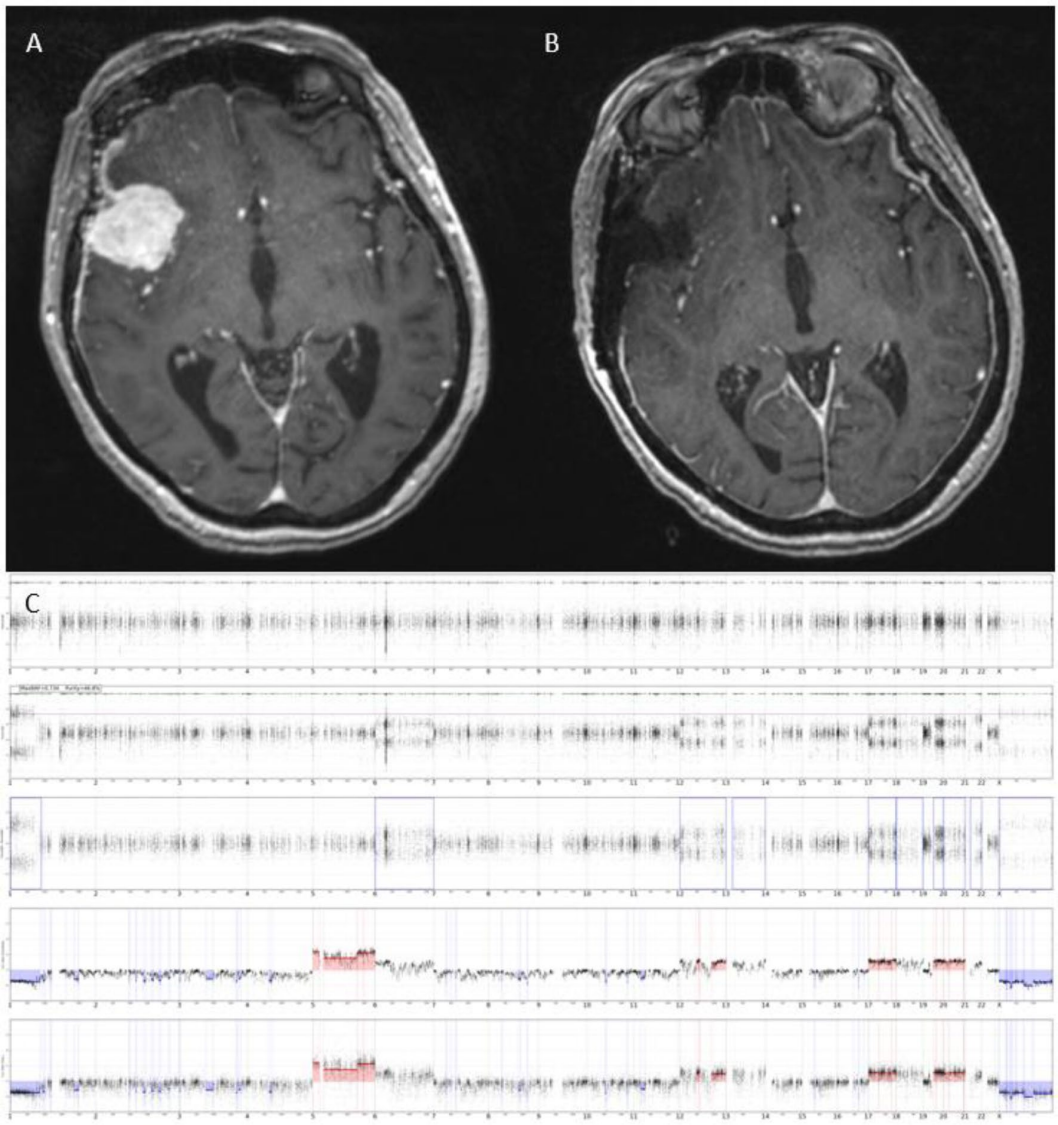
T1 MRI with contrast demonstrating an incidentally discovered right lateral sphenoid wing meningioma before (A) and after (B) gross total resection. Intraoperative pathology confirmed a WHO grade 1 meningioma. However, further genetic profiling of the tumor demonstrated a more aggressive genomic signature, with a high number of copy number variations (CNV) demonstrated on CNV plot (C). The postoperative course of this patient was uncomplicated and they were followed closely with surveillance imaging without receiving radiation therapy
